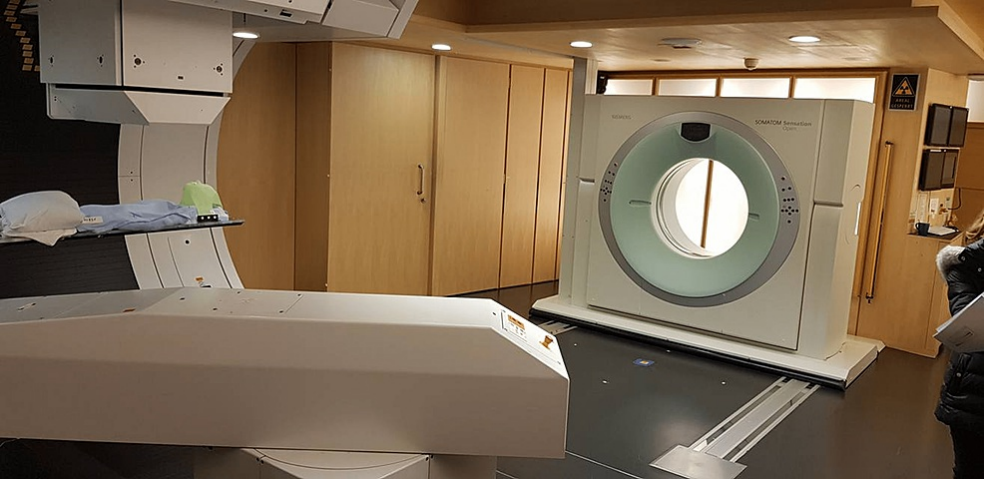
Gantry 2 with Computed Tomography on rails
